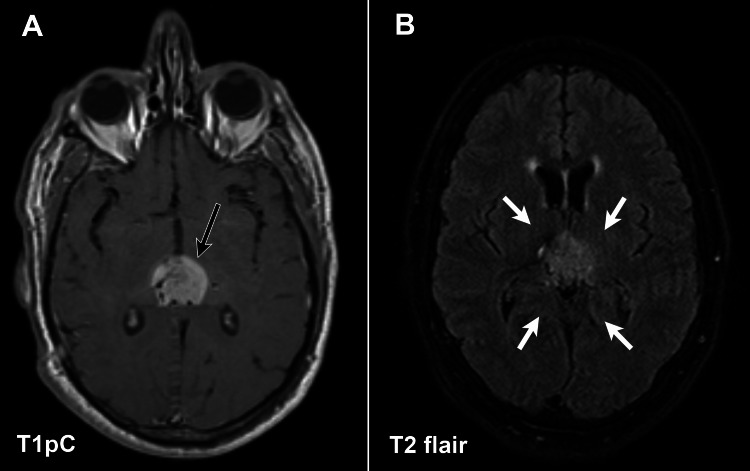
T1 post-contrast (A) and T2 flair (B) MRI at the initial presentation of a pineal glioblastoma. There is an approximate 25-mm enhancing pineal mass on T1 post-contrast imaging (black arrow), with no evidence of edema to the parenchyma highlighted by the white arrows that surround the lesion on T2 flair MRI.
